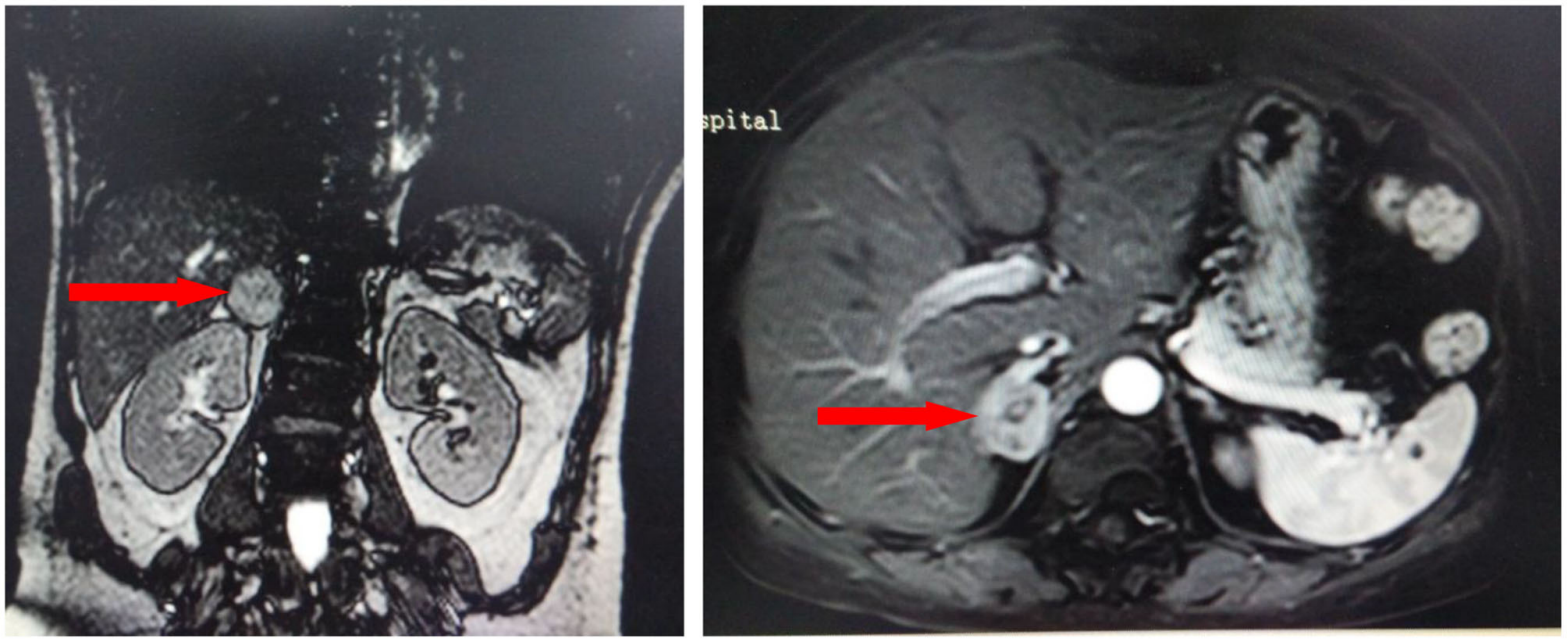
Contrast-enhanced computed tomography demonstrated an inhomogeneous right adrenal mass (2.5 × 3.0 cm, red arrows).
